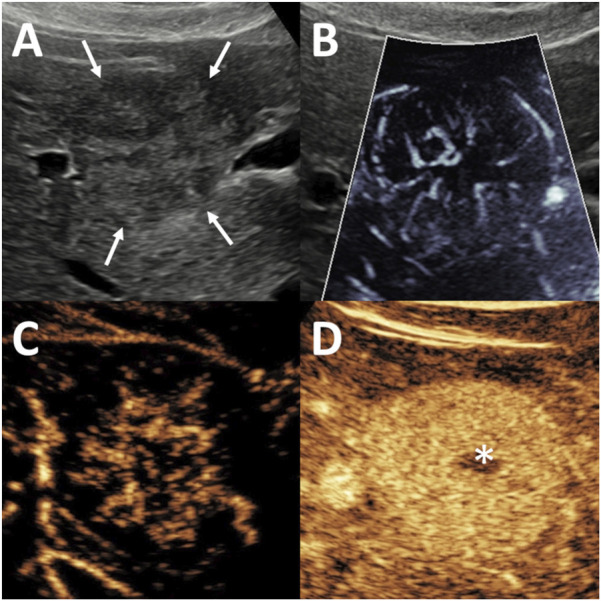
Evaluation of focal nodular hyperplasia (FNH) using microvascular imaging (MVFI) and contrast-enhanced ultrasound (CEUS). The lesion, measuring 20 mm in diameter, was located in segment 5, right beneath the liver capsule. (A) B-mode ultrasound (US) shows a nearly isoechoic lesion with poorly defined margins (arrows). (B) MVFI reveals a characteristic spoke-wheel vascular pattern. (C) In the early arterial phase of CEUS, the lesion demonstrates centrifugal enhancement with stellate vascularity. (D) During the venous phase, the lesion remains hyperenhancing relative to the surrounding liver, and a centrally located hypoenhancing scar (asterisk) is visible.
